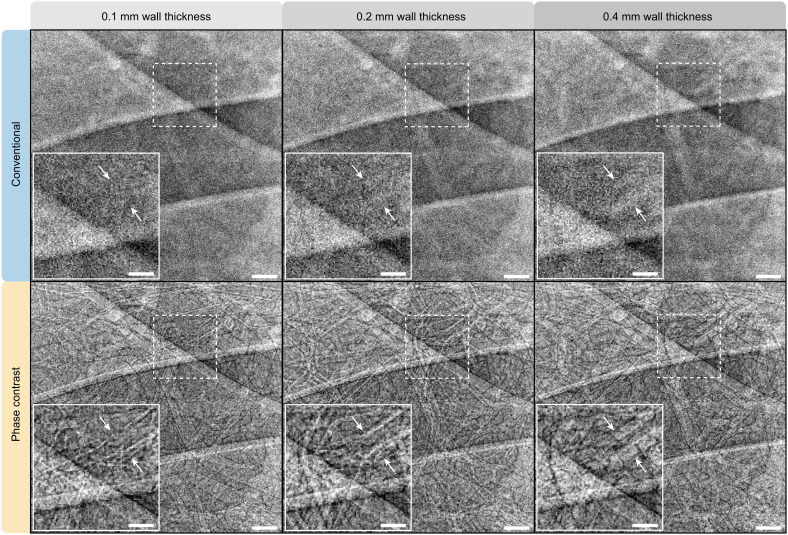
Visualizing wall thickening of small airways. Here, a smaller area (2 × 2 cm^2^) from a virtual chest radiograph is shown (*Top*, conventional; *Bottom*, phase contrast at *z* = 12 m) with increasingly thicker airway walls: 0.1 mm, 0.2 mm, and 0.4 mm. The insets (*Bottom Left*) of each panel are zoom-ins of an airway (indicated by the arrows) with the walls clearly visualized in the phase-contrast radiograph. The pixel size in all images is 50 μm. (Scale bars, 2 mm (overview), 1 mm (*inset*).)
